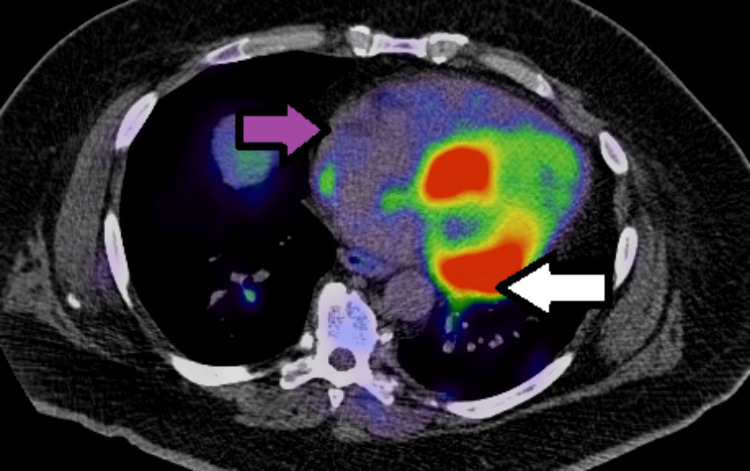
Cardiac PET CT Nodular SUV uptake within the myocardium (white arrow); No SUV uptake (purple arrow). SUV: Standardized uptake value
